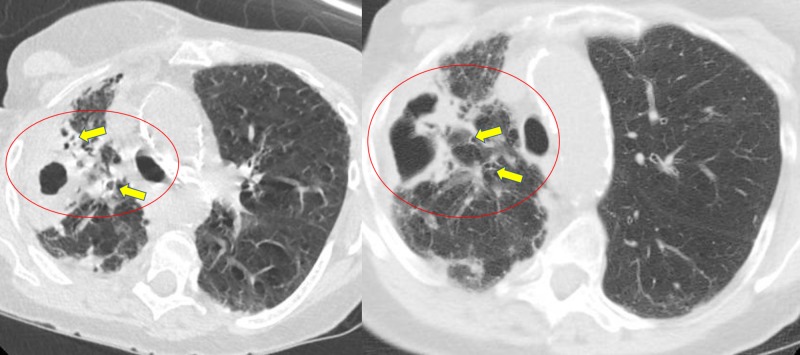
Initial axial computed tomography of chest showing right upper lobe cavitary opacity (circled) with adjacent bronchiectasis (yellow arrows)
